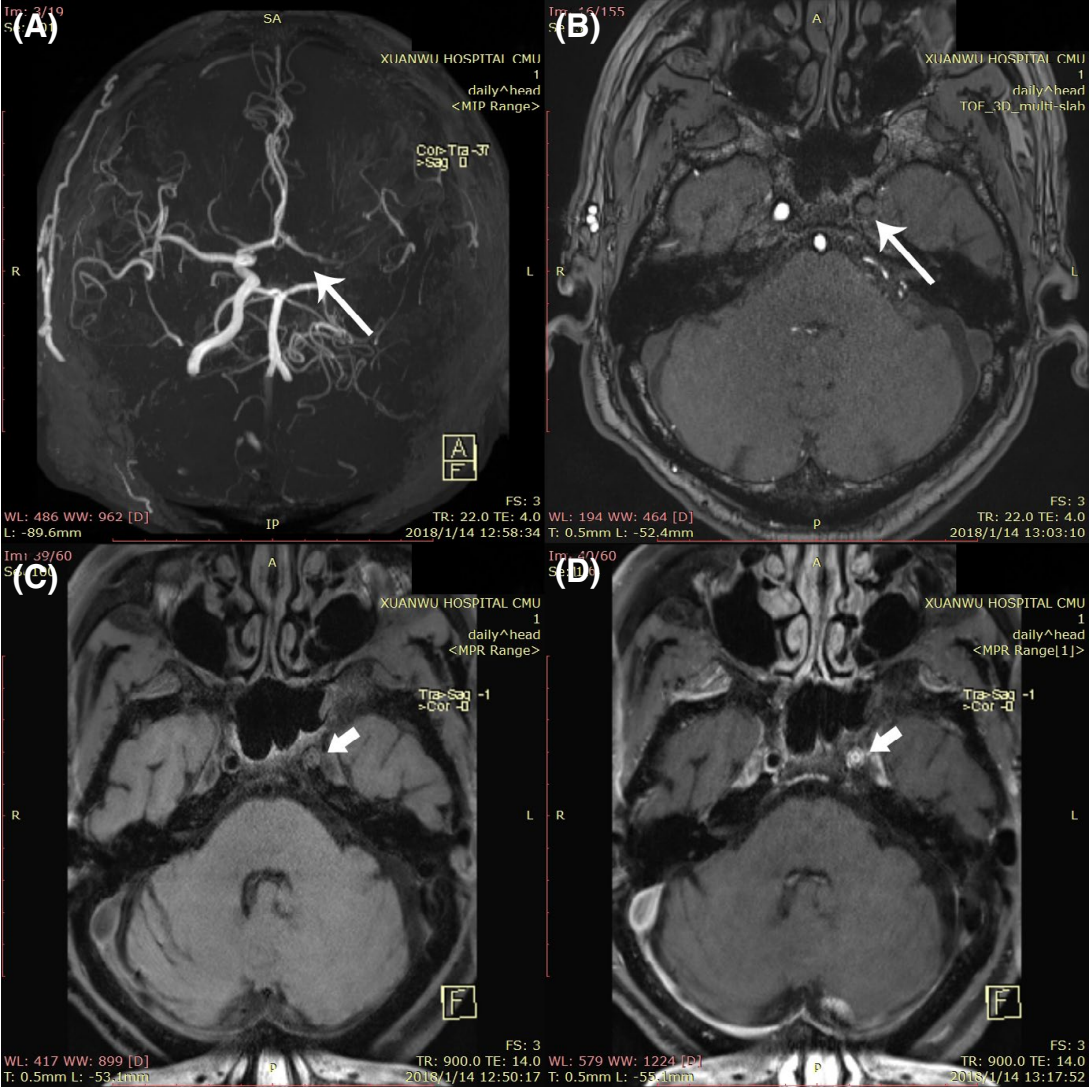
Features of moyamoya vessels on TOF MRA and HR‐MRI map. TOF MRA shows (A) cross‐sectional image showed moyamoya vessel, (B) the left internal carotid artery with complete occlusion (long arrow), (C) T1‐weighted HR‐MRI, and (D) contrast‐enhanced HR‐MRI present the concentric inward remodeling (short arrow) in the left internal carotid artery lumen
